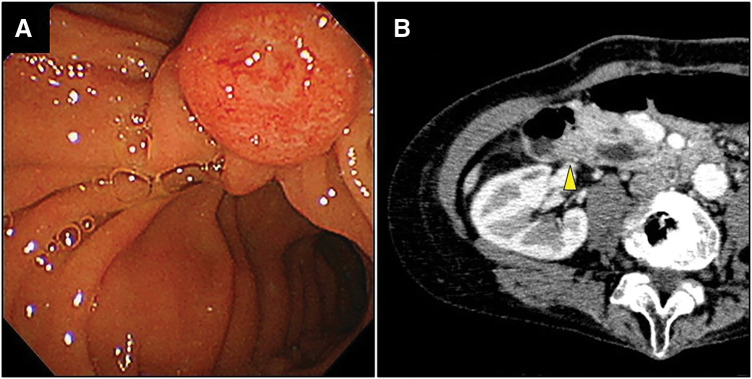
Preoperative findings. (A) Upper gastrointestinal endoscopy revealed an enlarged duodenal main papilla. (B) CECT revealed a 13-mm contrast-enhanced nodule in the duodenal papillary region (a yellow arrowhead). CECT, contrast-enhanced computed tomography
